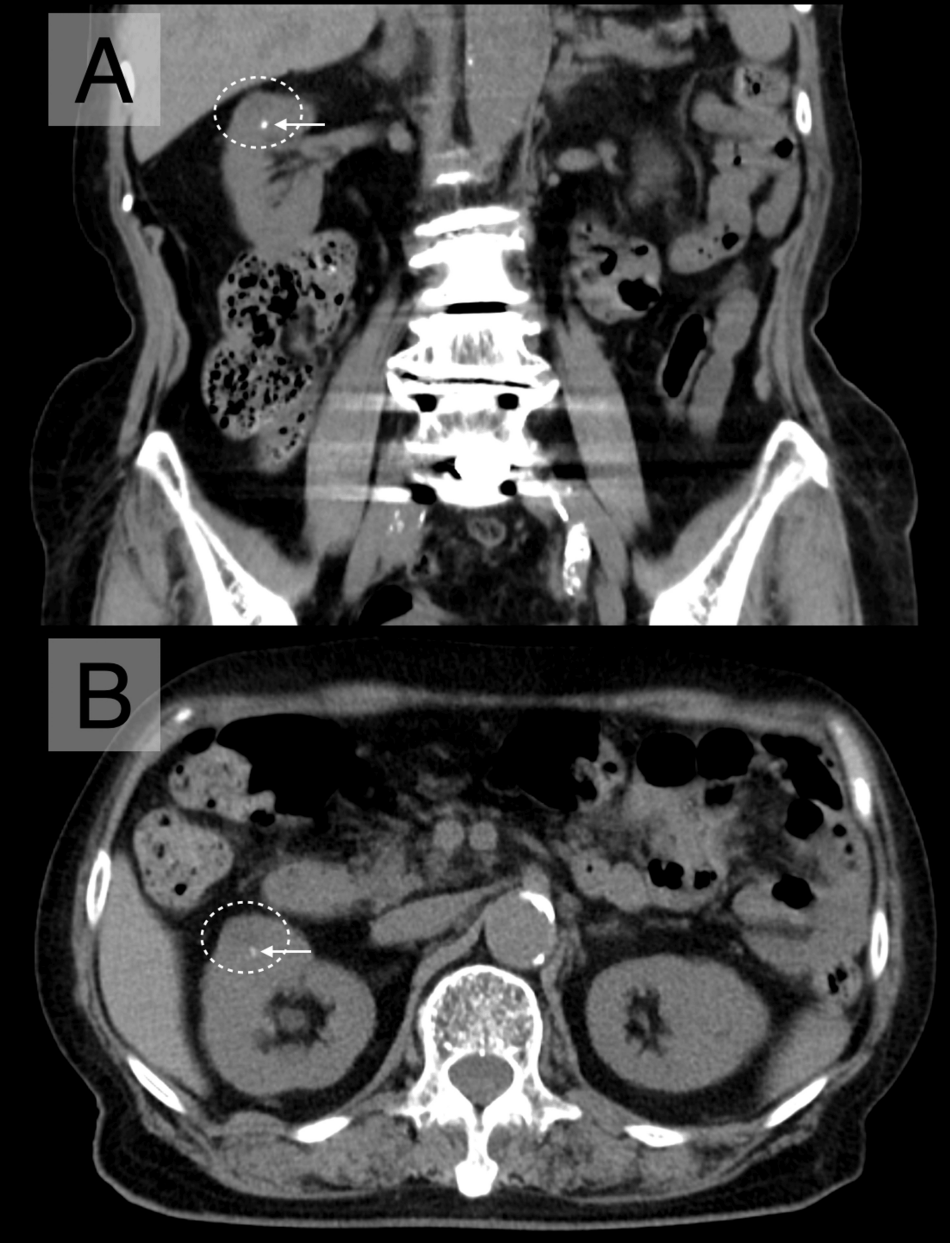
Abdominal CT six months before admission. An abdominal CT performed six months before admission showed a 10-mm solitary right renal cyst (dotted circles) with fine wall calcification (arrow), classified as Bosniak category II. (A) Coronal and (B) axial views.
